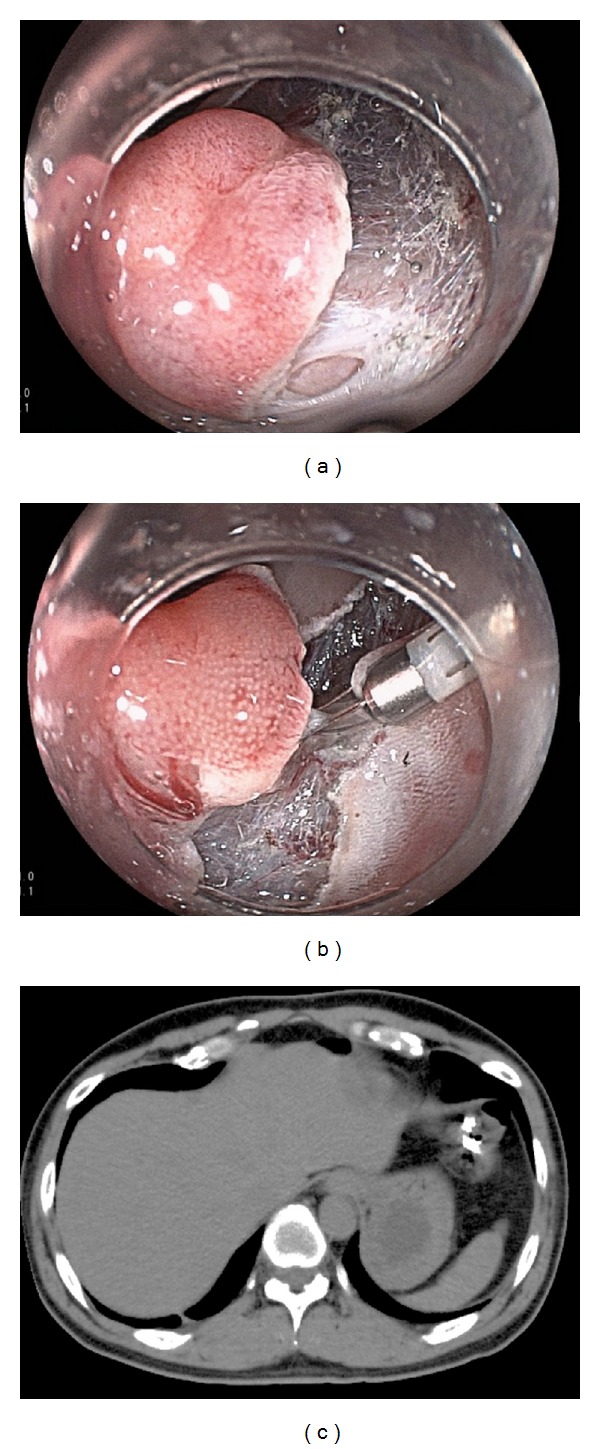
(a) Perforation during dissection. (b) The perforation was closed by endoscopic clipping. (c) Computed tomography revealed the presence of free air outside of the colorectum.
